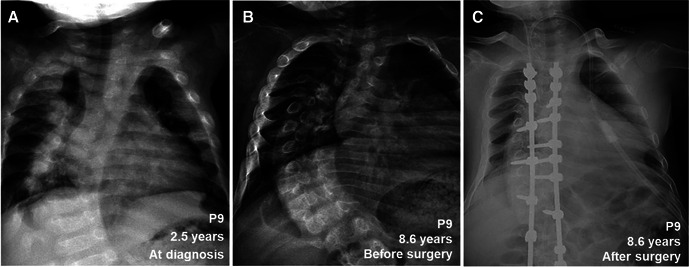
Comparison of skeletal x-ray images of VDDR1A patient P9 at diagnosis and after treatment. (A) At diagnosis, (B) after treatment was discontinued, (C) after surgery.
